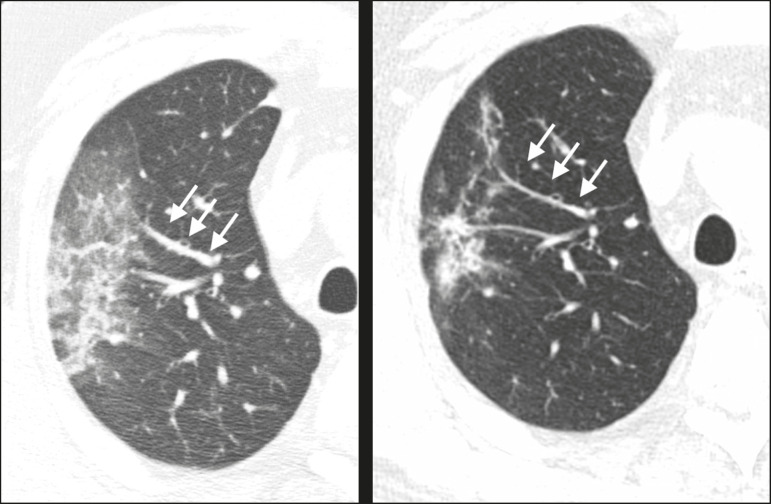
A 64-year-old male patient. A: CT scan acquired on day 3 after symptom onset. Note the increased vessel caliber in the parenchyma of the right upper lobe (arrows), adjacent to an area with the crazy-paving pattern. B: CT scan, acquired 28 days after the first CT, showing reduced vessel caliber and resolution of the crazy-paving pattern, with small residual consolidation.
